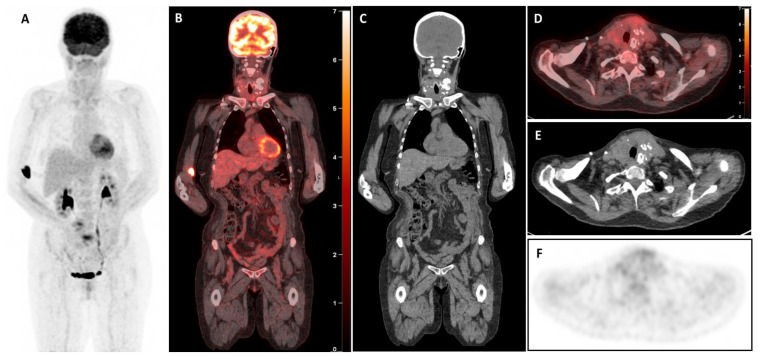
Interim [^18^F]FDG-PET/CT images after four cycles of chemotherapy demonstrated normal tracer distribution on the whole-body MIP image (A), with diffuse axial and appendicular skeletal uptake consistent with reactive post-chemotherapy changes, and no abnormal tracer activity was noted in the neck region on the coronal projection (B,C). Notably, the [^18^F]-FDG PET/CT revealed no pathological uptake in the residual thyroid mass (SUVmax 2.7) (D–F), lesser than the liver blood pool (SUVmax 3.1) consistent with a complete metabolic response (Deauville score 3).
